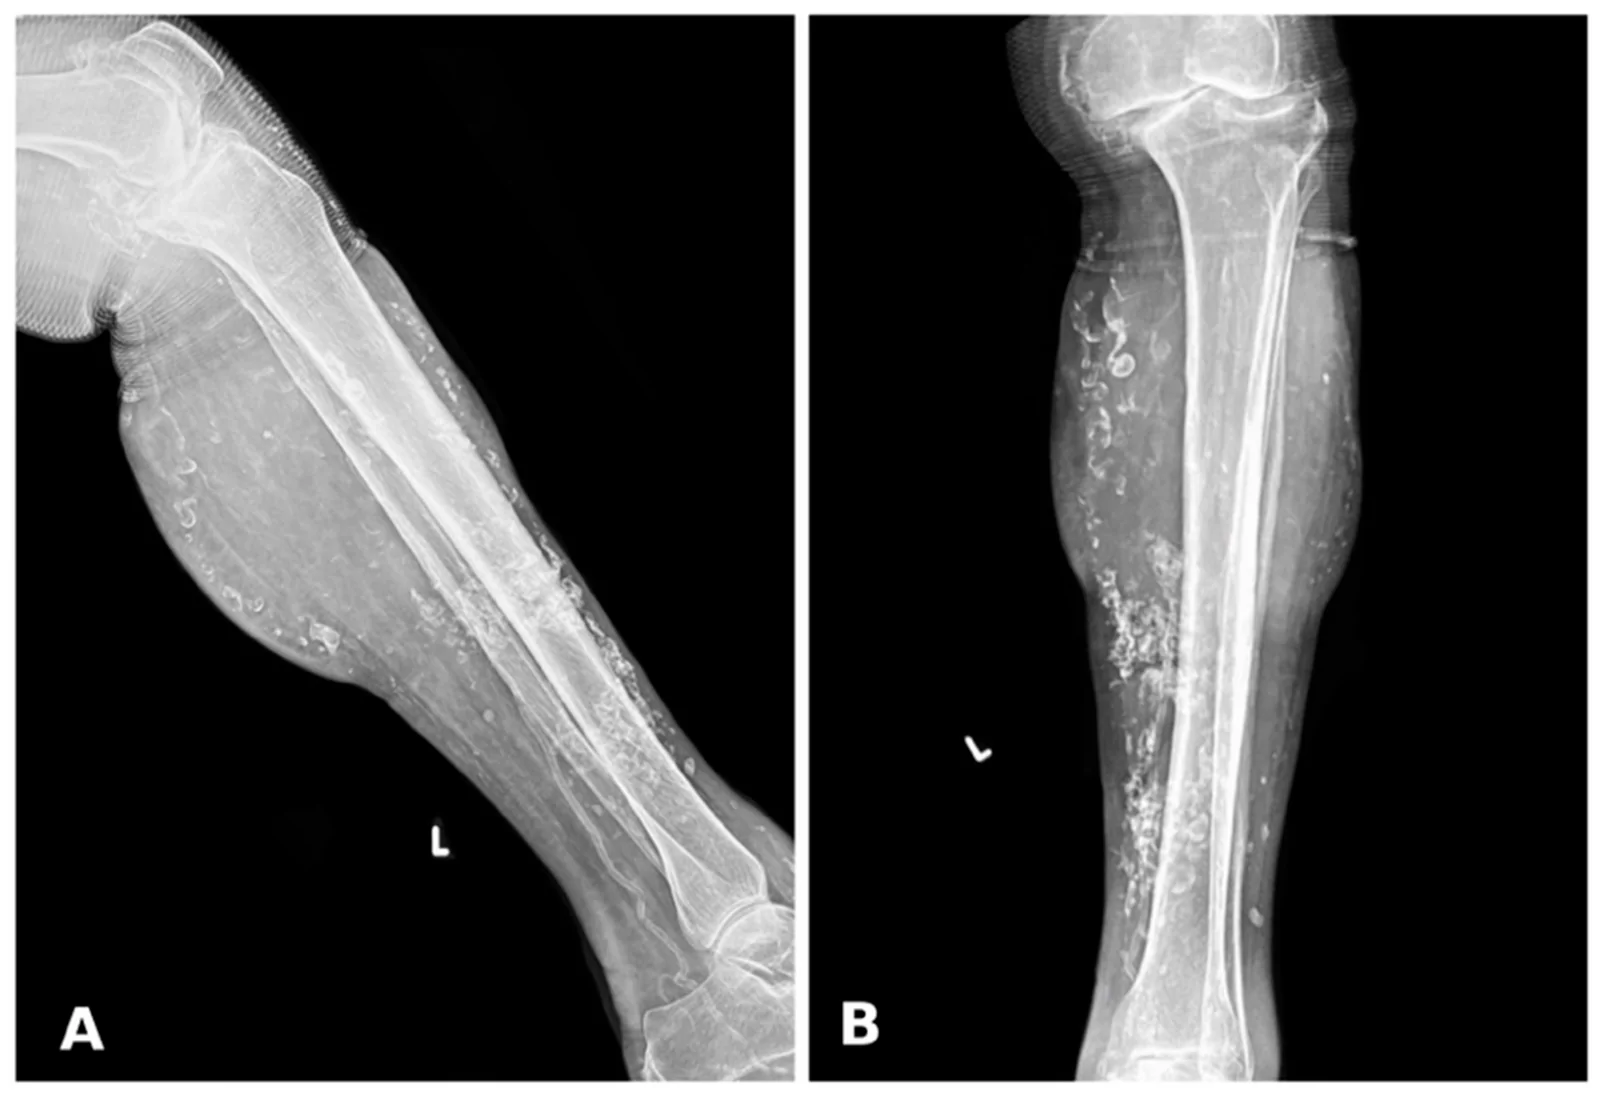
Radiographs of dystrophic calcinosis cutis of the lower left limb. Oblique (A) and anteroposterior (B) projections show multiple irregular calcifications distributed throughout the subcutaneous tissues. L: left.
